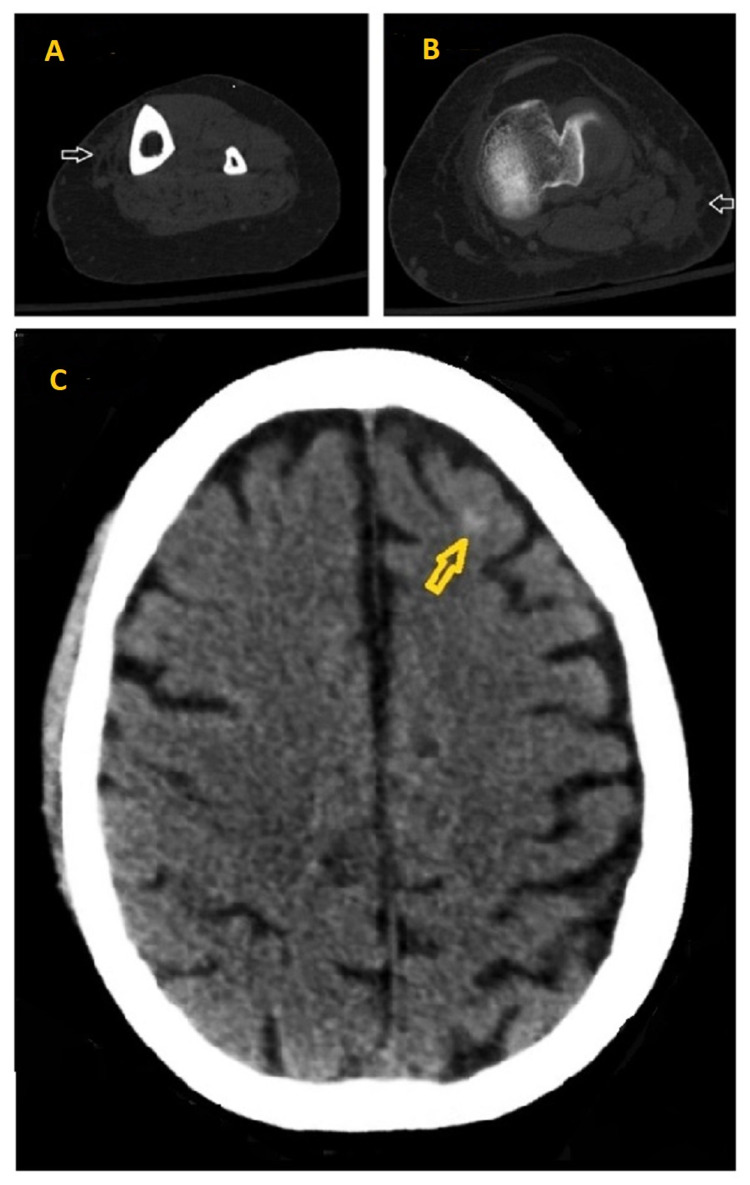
Initial CT findings 1A, 1B: CT left lower extremity showing stranding (white arrows) in the subcutaneous fat overlying the anteromedial aspect of the tibia and the posterolateral lower third of the femur respectively. 1C: CT head without IV contrast showing left frontal subarachnoid hemorrhage (yellow arrow).
